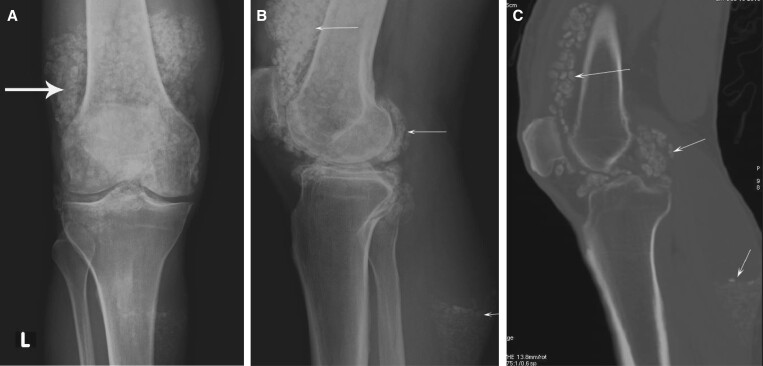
Synovial chondromatosis: (A) and (B) AP and lateral projection radiographs showing extensive loose bodies. (C) Corresponding CT image showing loose bodies.
